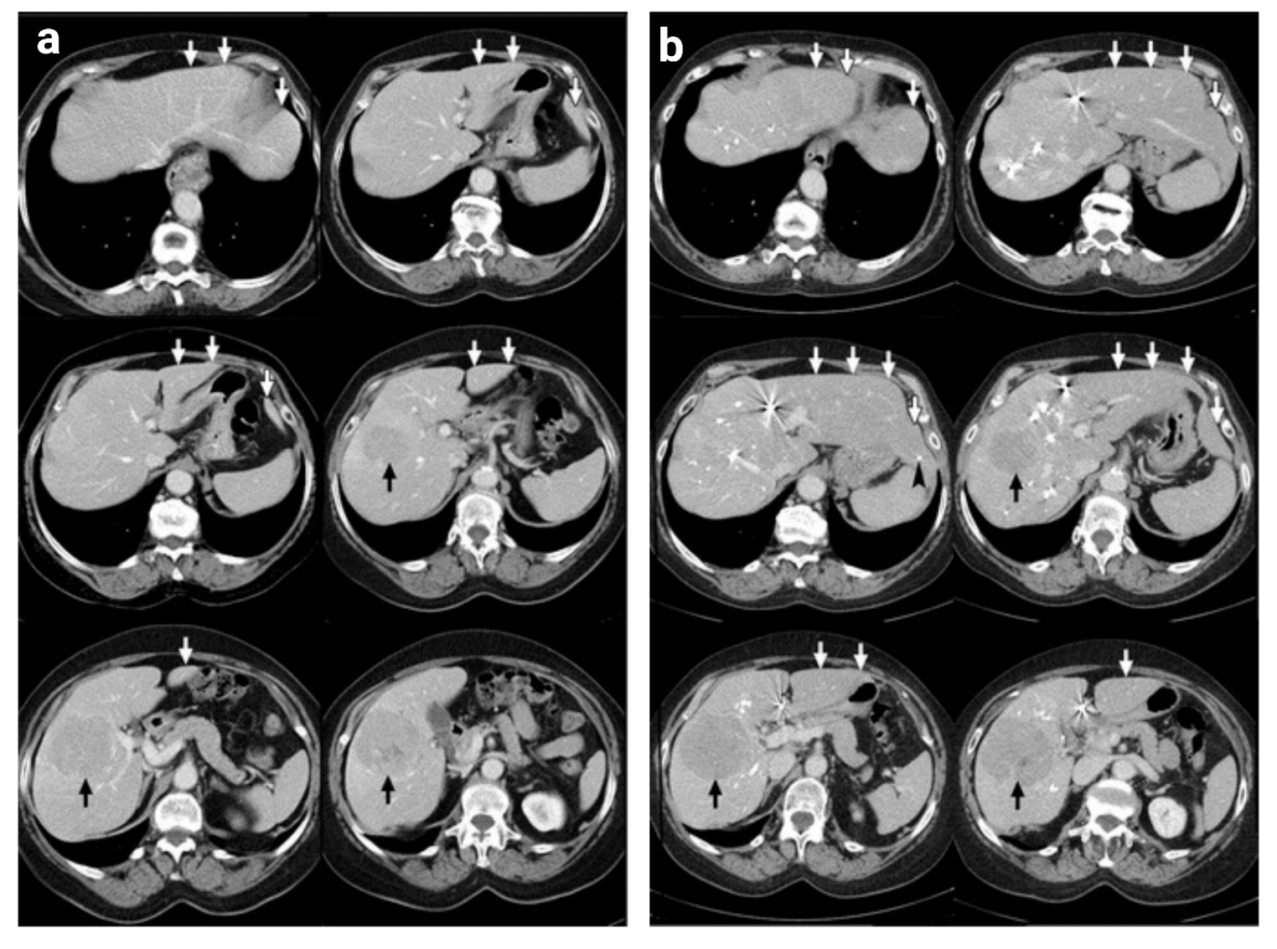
Liver growth after PVESA in a patient with HCC. (a) Axial helical computed tomography scans before and (b) 14 days after PVE and intraportal autologous CD133 + BMSC application revealed marked growth of segments II and III, marked by white arrows (black arrowhead, dislocated cyanoacrylate-to-iodinized oil particle; black arrow, hepatocellular carcinoma). Images have been adapted with permission from Fürst et al. 2007 [87]. The figure has been created using Biorender.com
